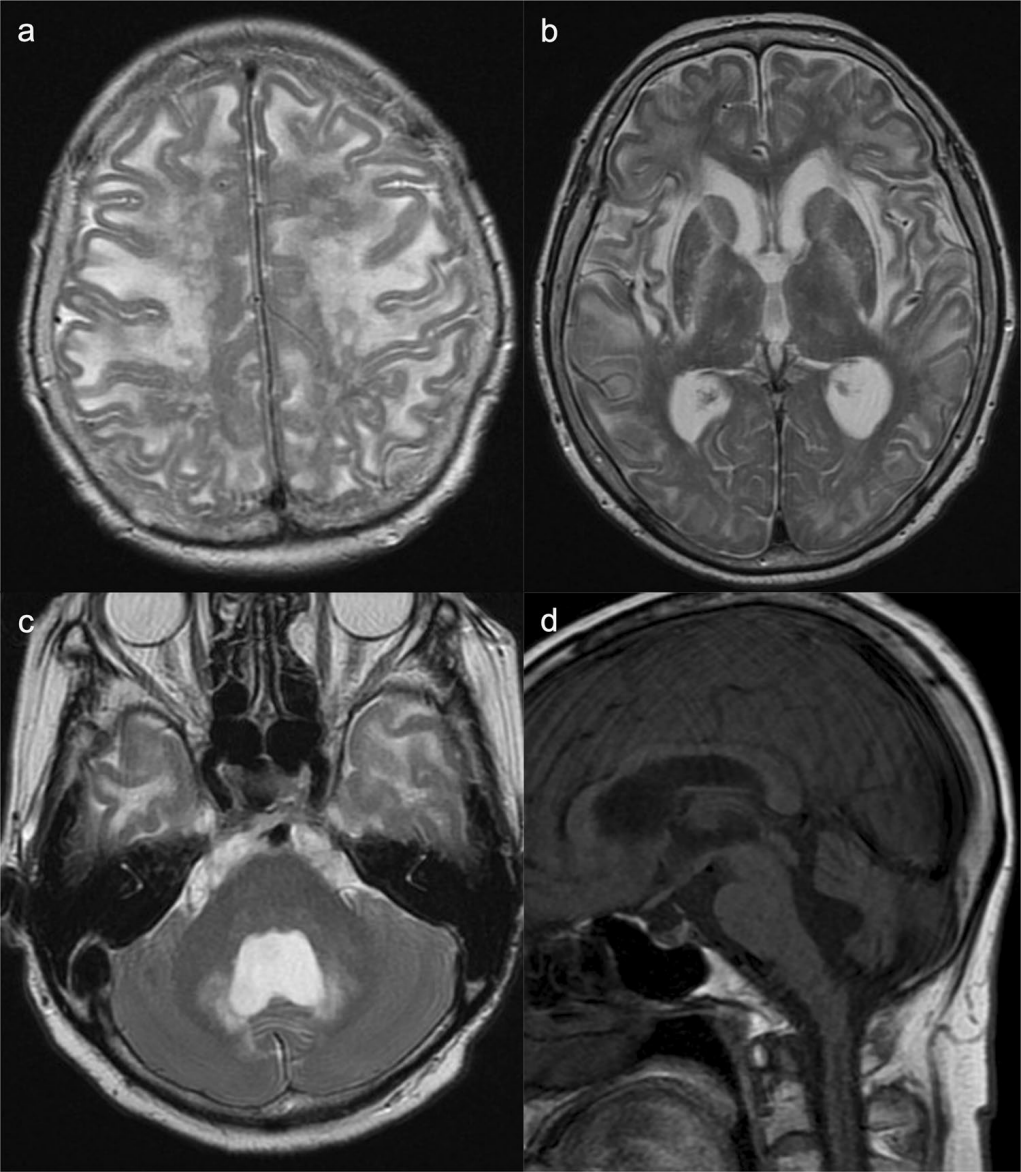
A 69-year-old, female patient with L-2-hydroxyglutaric aciduria (L2HGA). Mental retardation was present since early childhood. She had a resting tremor of the left upper extremity, gait disturbance, and involuntary movements. a–c T2-weighted images demonstrated increased signal intensity in the subcortical white matter, anterior limb of the internal capsules, globi pallidi, external capsules, and dentate nuclei. d Sagittal T1-weighted image showed cerebellar atrophy
